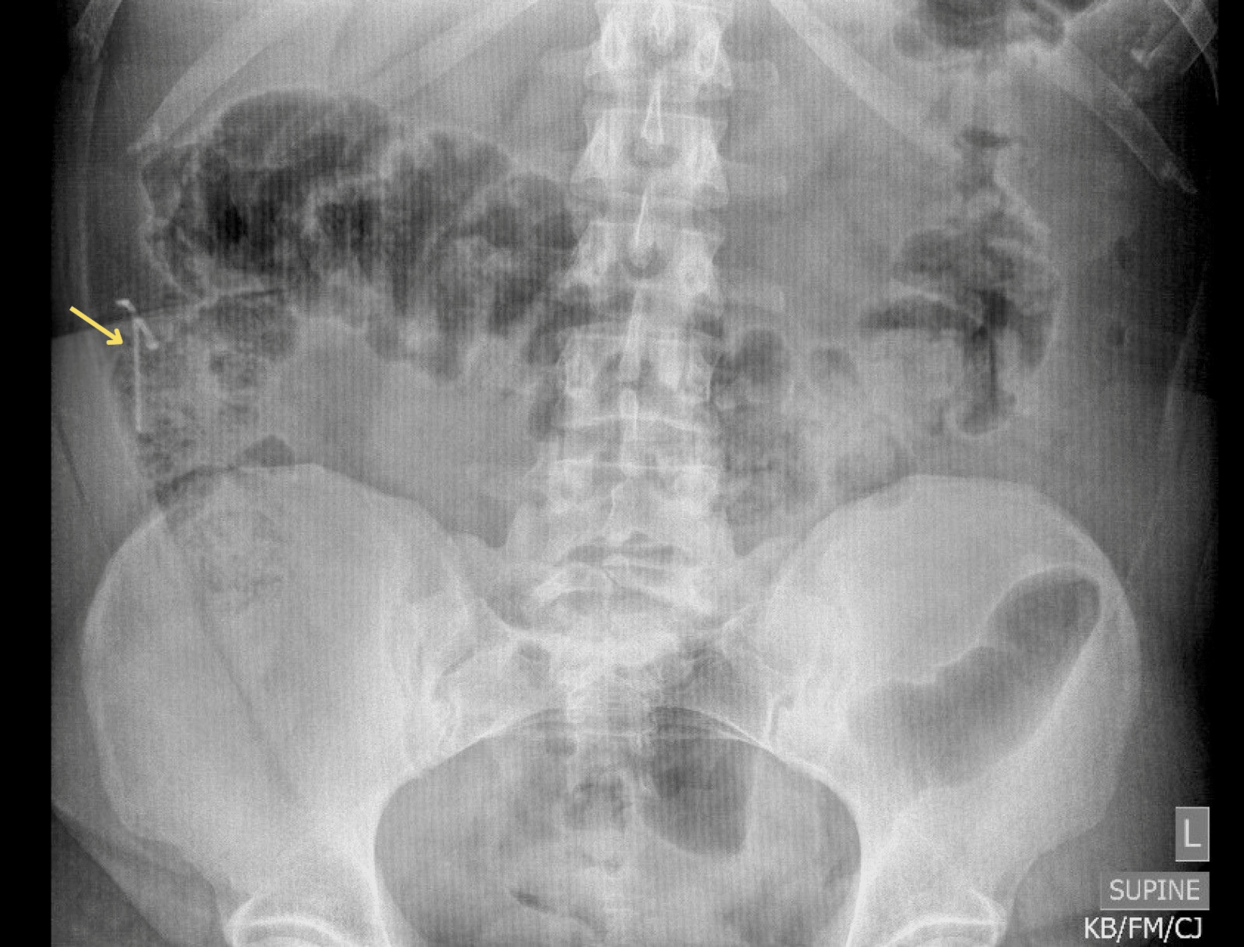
Plain radiograph of the abdomen and pelvis with the yellow arrow denoting an intra-abdominal foreign body
